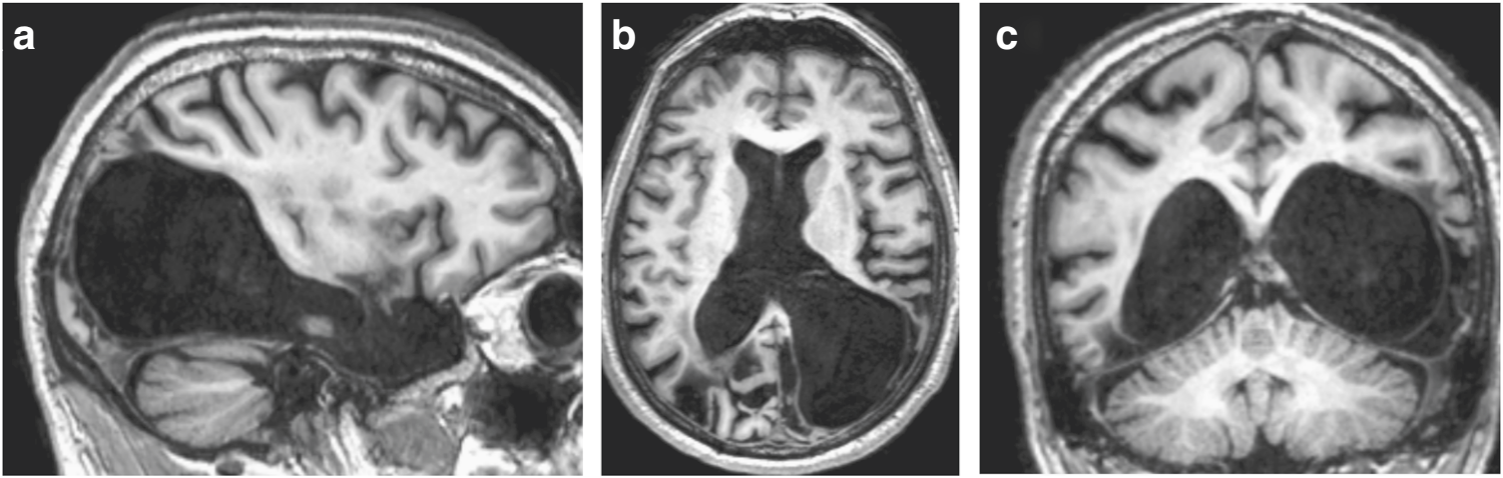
3T anatomical MRI scans of MS’s brain in 2018. Images show the sagittal (a), horizontal (b), and coronal (c) planes of the brain. The left hemisphere has damage to the temporal and occipital lobes, but the parietal and frontal lobes are largely preserved. The right hemisphere damage is much more substantial. The dorsal part of both hemispheres is intact. An extensive and detailed description of MS’s lesion can be found elsewhere^41^
